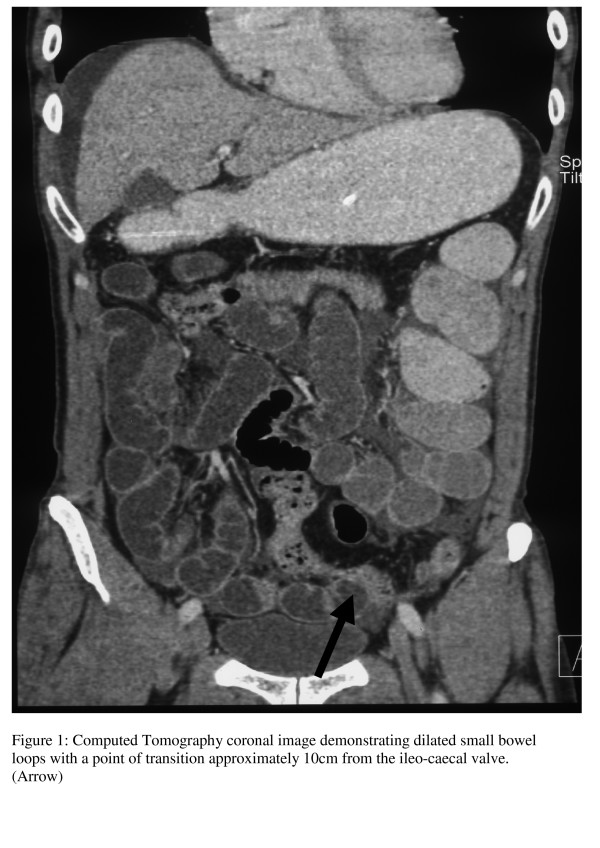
Coronal CT demonstrating dilated small bowel with a transition point 10 cm from the ileocaecal valve. (Arrow).
